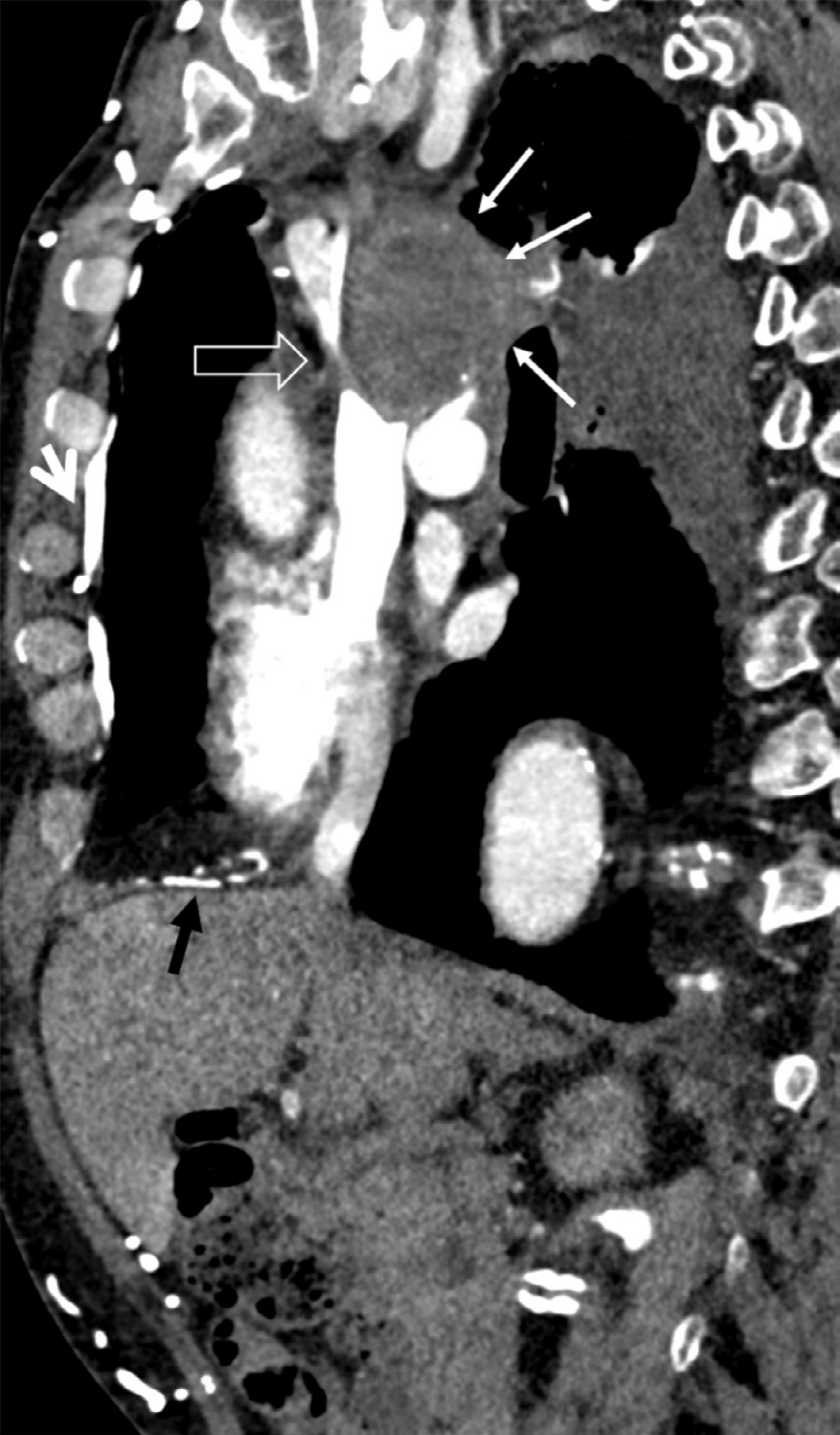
Sagittal CT showing mediastinal mass and SVC occlusion.
